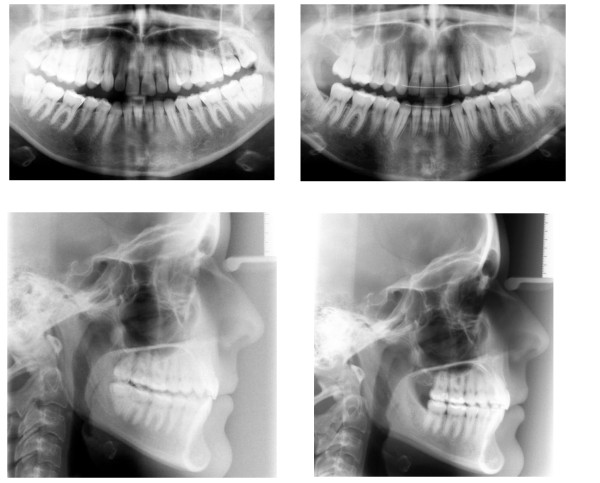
Comparison of the pre- (left) and the post-treatment xrays (right).
